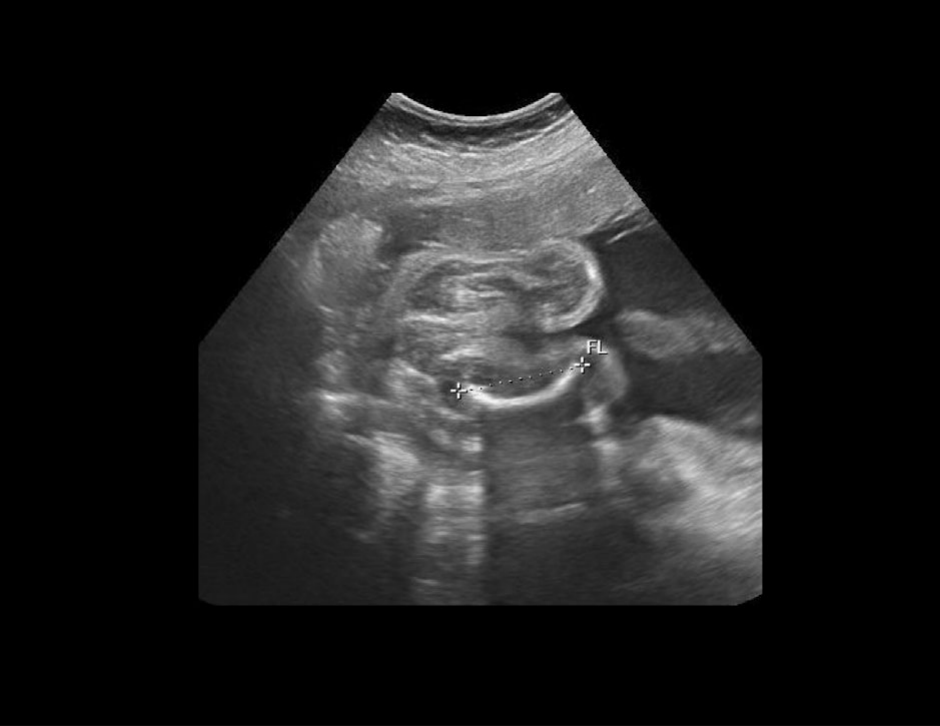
Prenatal ultrasound at 22 weeks showing bowing of the femur (the crosshairs show the extremities of the femur).
